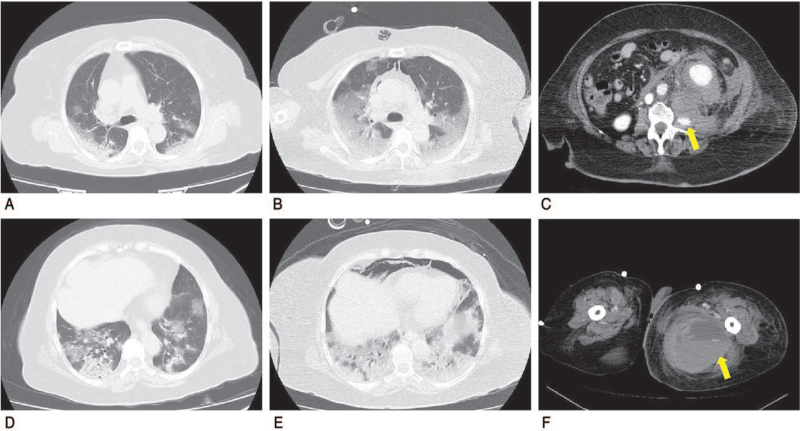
Computed tomography (CT) findings at the bifurcation and the lung base, and contrast-enhanced CT findings. (A) Chest CT performed on day 1 showed extensive ground-glass opacity (GGO). (B) Chest CT performed on day 6 showed further enlargement of the GGO. (C) The contrast-enhanced CT venous phase image obtained on day 13 after admission revealed active bleeding with extravasation of the left retroperitoneal space (arrow). Transcatheter arterial embolization (TAE) was performed using primary embolization material for the L2–L3 left lumbar artery. (D) The contrast-enhanced CT venous phase image obtained on day 28 after admission revealed active bleeding with extravasation in the left thigh muscle (arrow). TAE was immediately performed using primary embolization material for the left deep femoral artery, but extravasation was not clear on femoral artery angiography.
